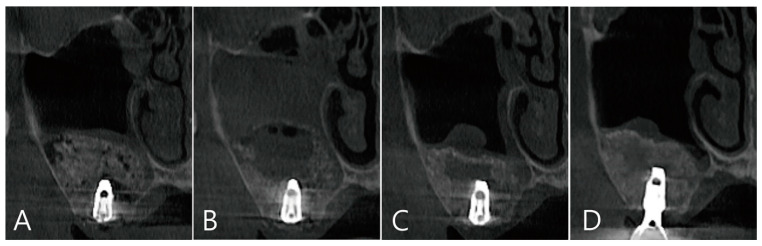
(A) Coronal image of the CBCT taken immediately after surgery. There was no perforation of the maxillary sinus membrane and no leakage of the bone graft substitute; (B) coronal image of the CBCT taken one week after surgery. A severely enlarged grafting void was observed above the implant apex, and the ostium was obstructed due to severe membrane thickening; (C) coronal image of the CBCT taken 6 months after surgery. Although the grafting void was reduced in size, it appears similar to the SCC; (D) the radiopacity of the removed grafting void site was increased in the CBCT images taken 6 months after the prosthesis was delivered.
